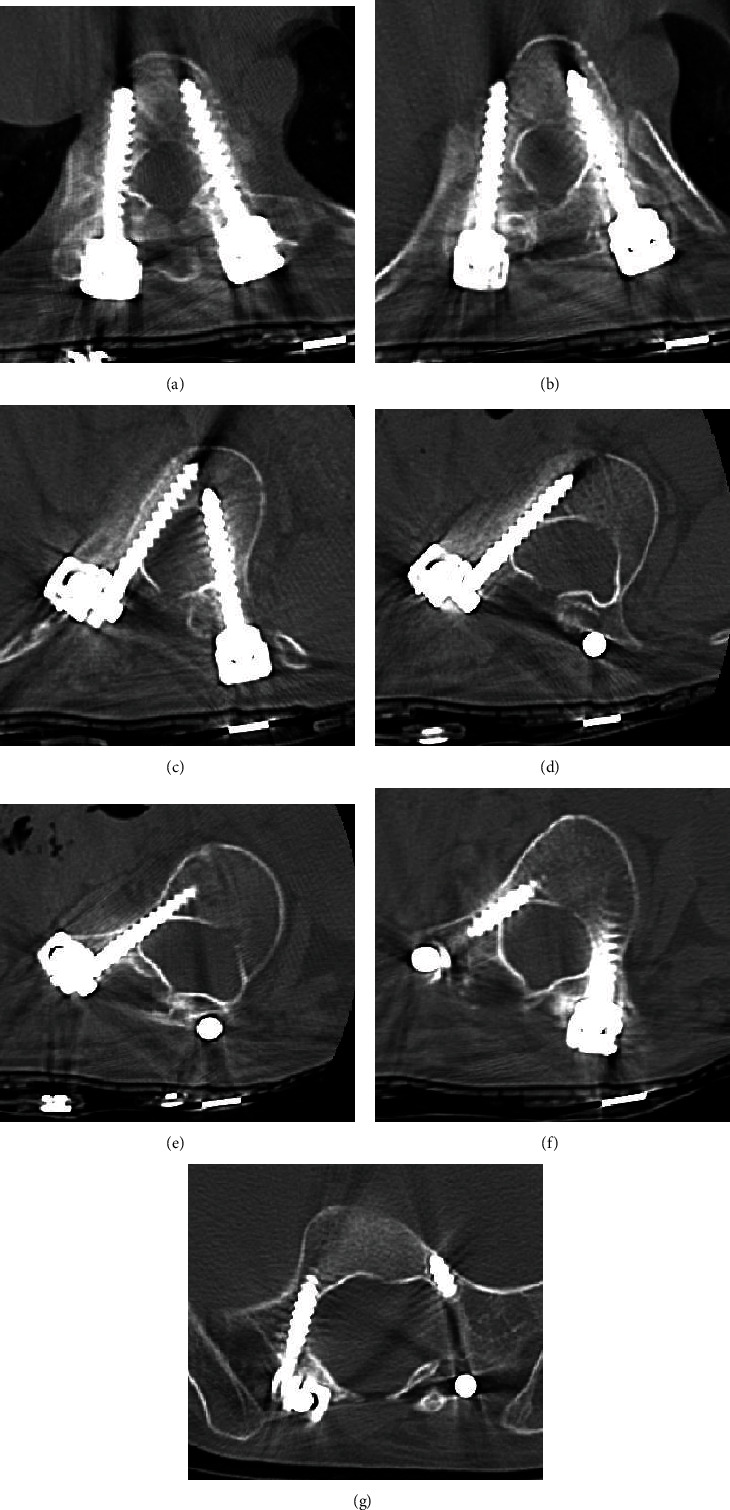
Postoperative axial CT: (a) T9, (b) T10, (c) L1, (d) L2, (e) L3, (f) L4, and (g) S1.
